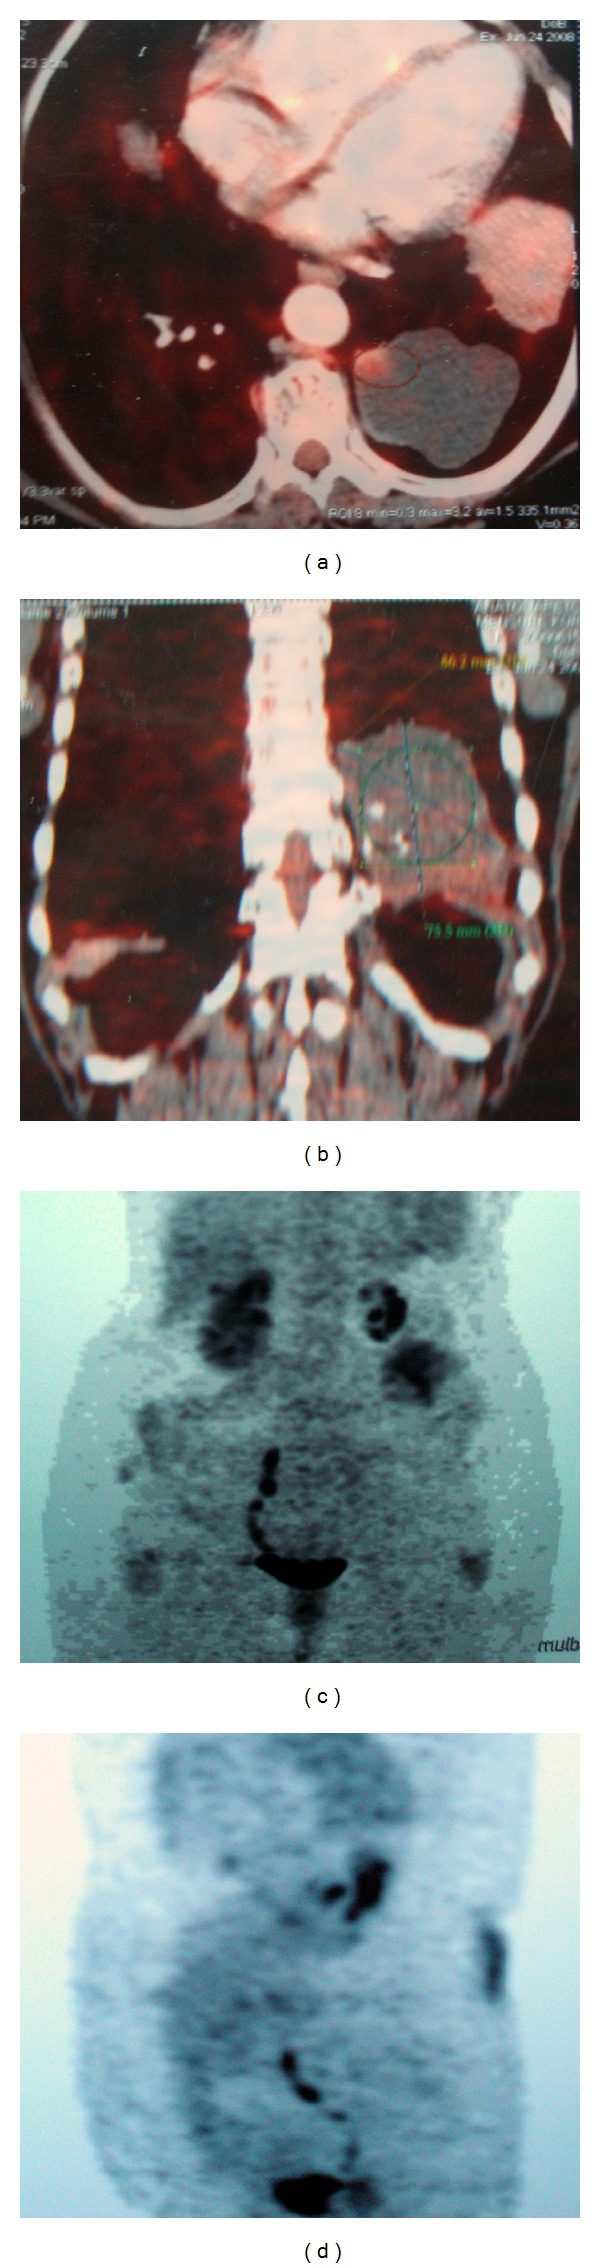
(a and b) Focally increased glycolytic activity in the lesion detected on PET CT. (c and d) Decreased glycolytic activity of the left kidney was seen on PET CT.
